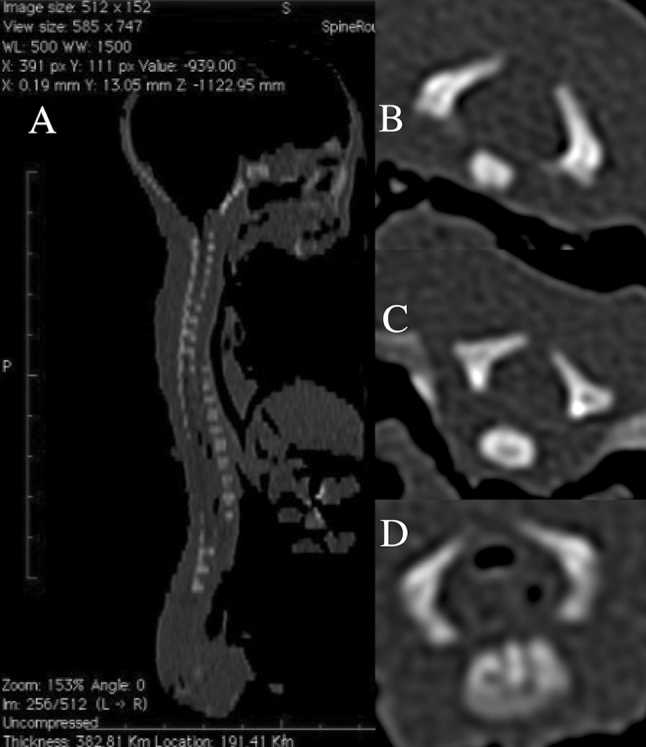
CT of a female fetus aged 24 weeks (in the sagittal projection) recorded in DICOM formats (a) with spinal ossification centers (in the transverse projection) of C4 (b), T6 (c), and L3 (d) vertebrae, being assessed by Osirix 3.9
